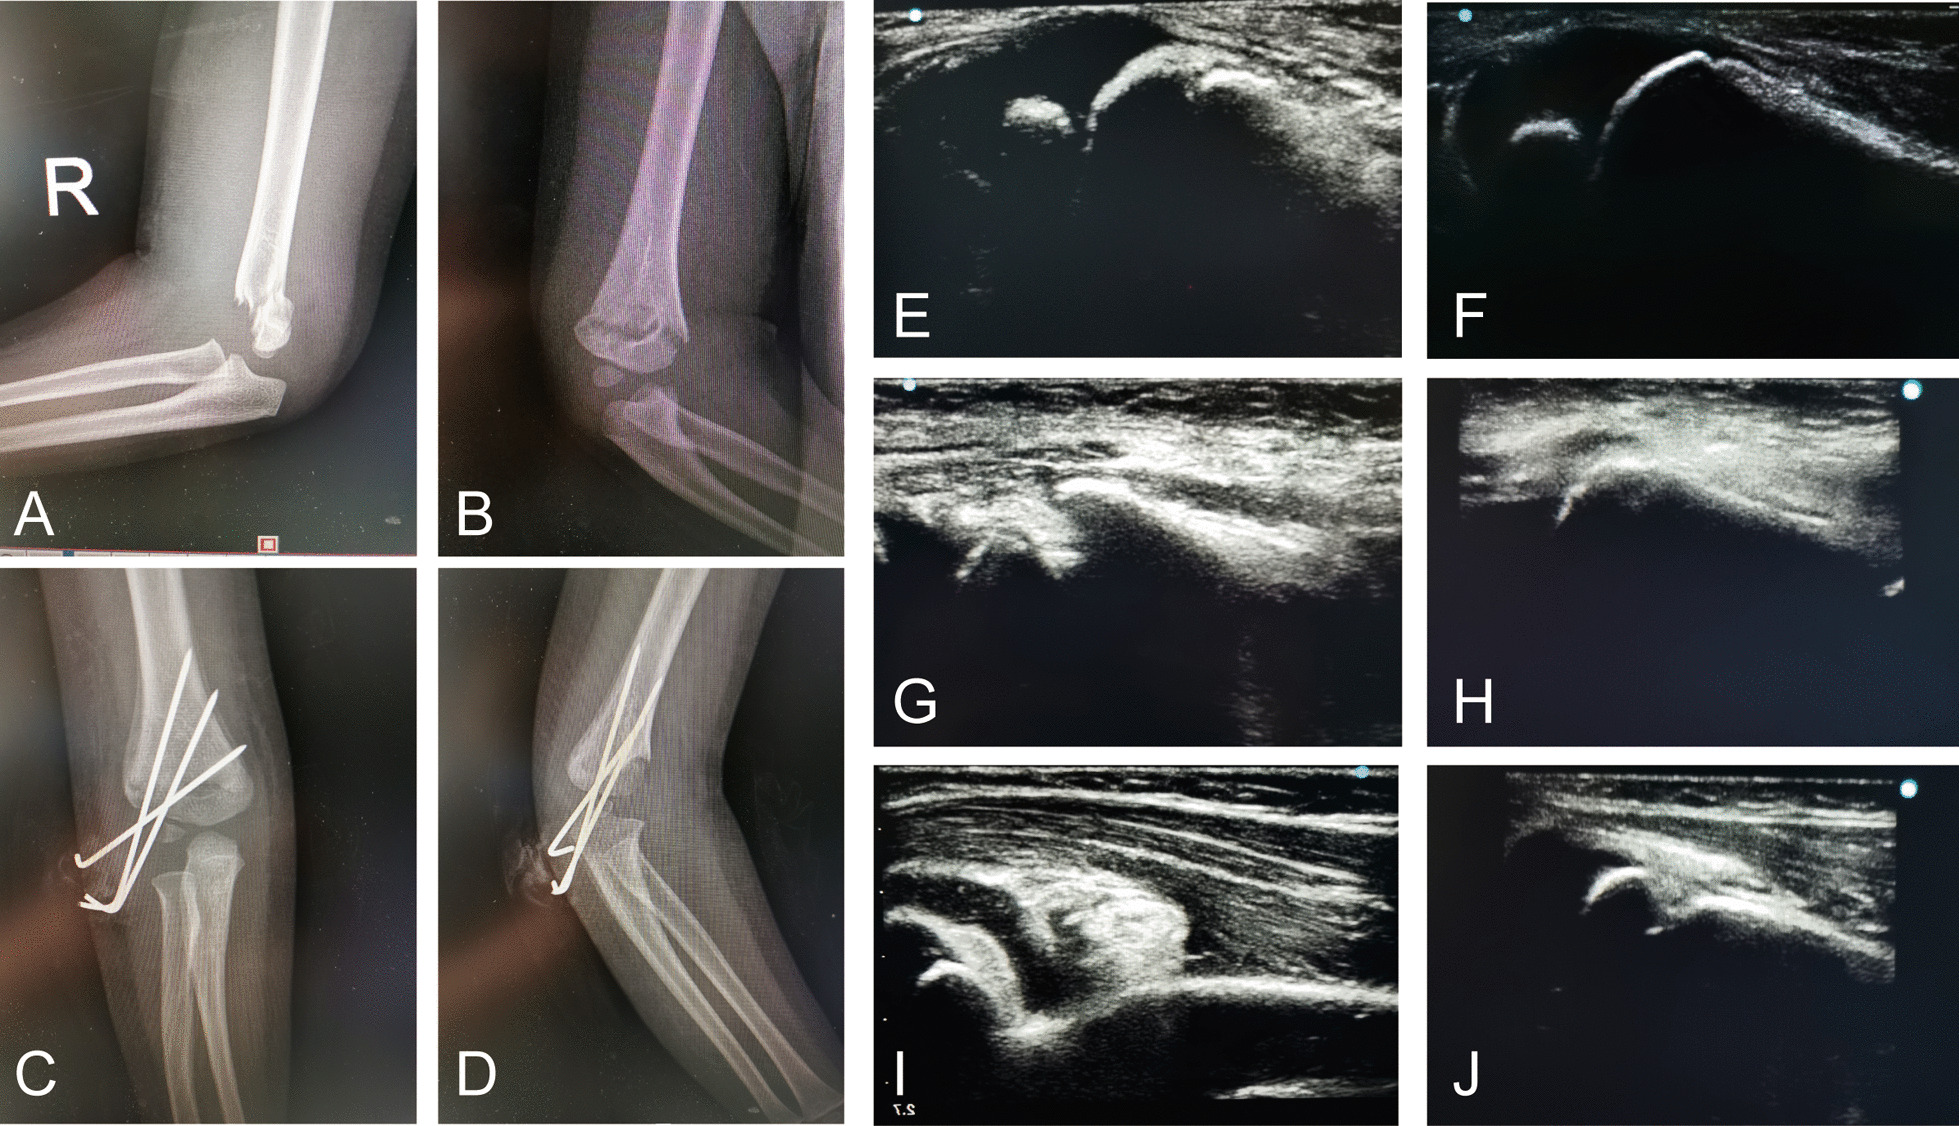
a Preoperative lateral radiogram of the patient with Gartland II humeral fracture; b Preoperative anteroposterior radiogram. c Postoperative anteroposterior radiogram after CRPP; d Postoperative lateral radiogram after CRPP. e Lateral, g medial and i posterior sonogram of forearm shows softtissue swelling around elbow and medial displacement of distal humerus; f Lateral, h medial and j posterior sonogram after reduction shows distal humerus back in its normal position
